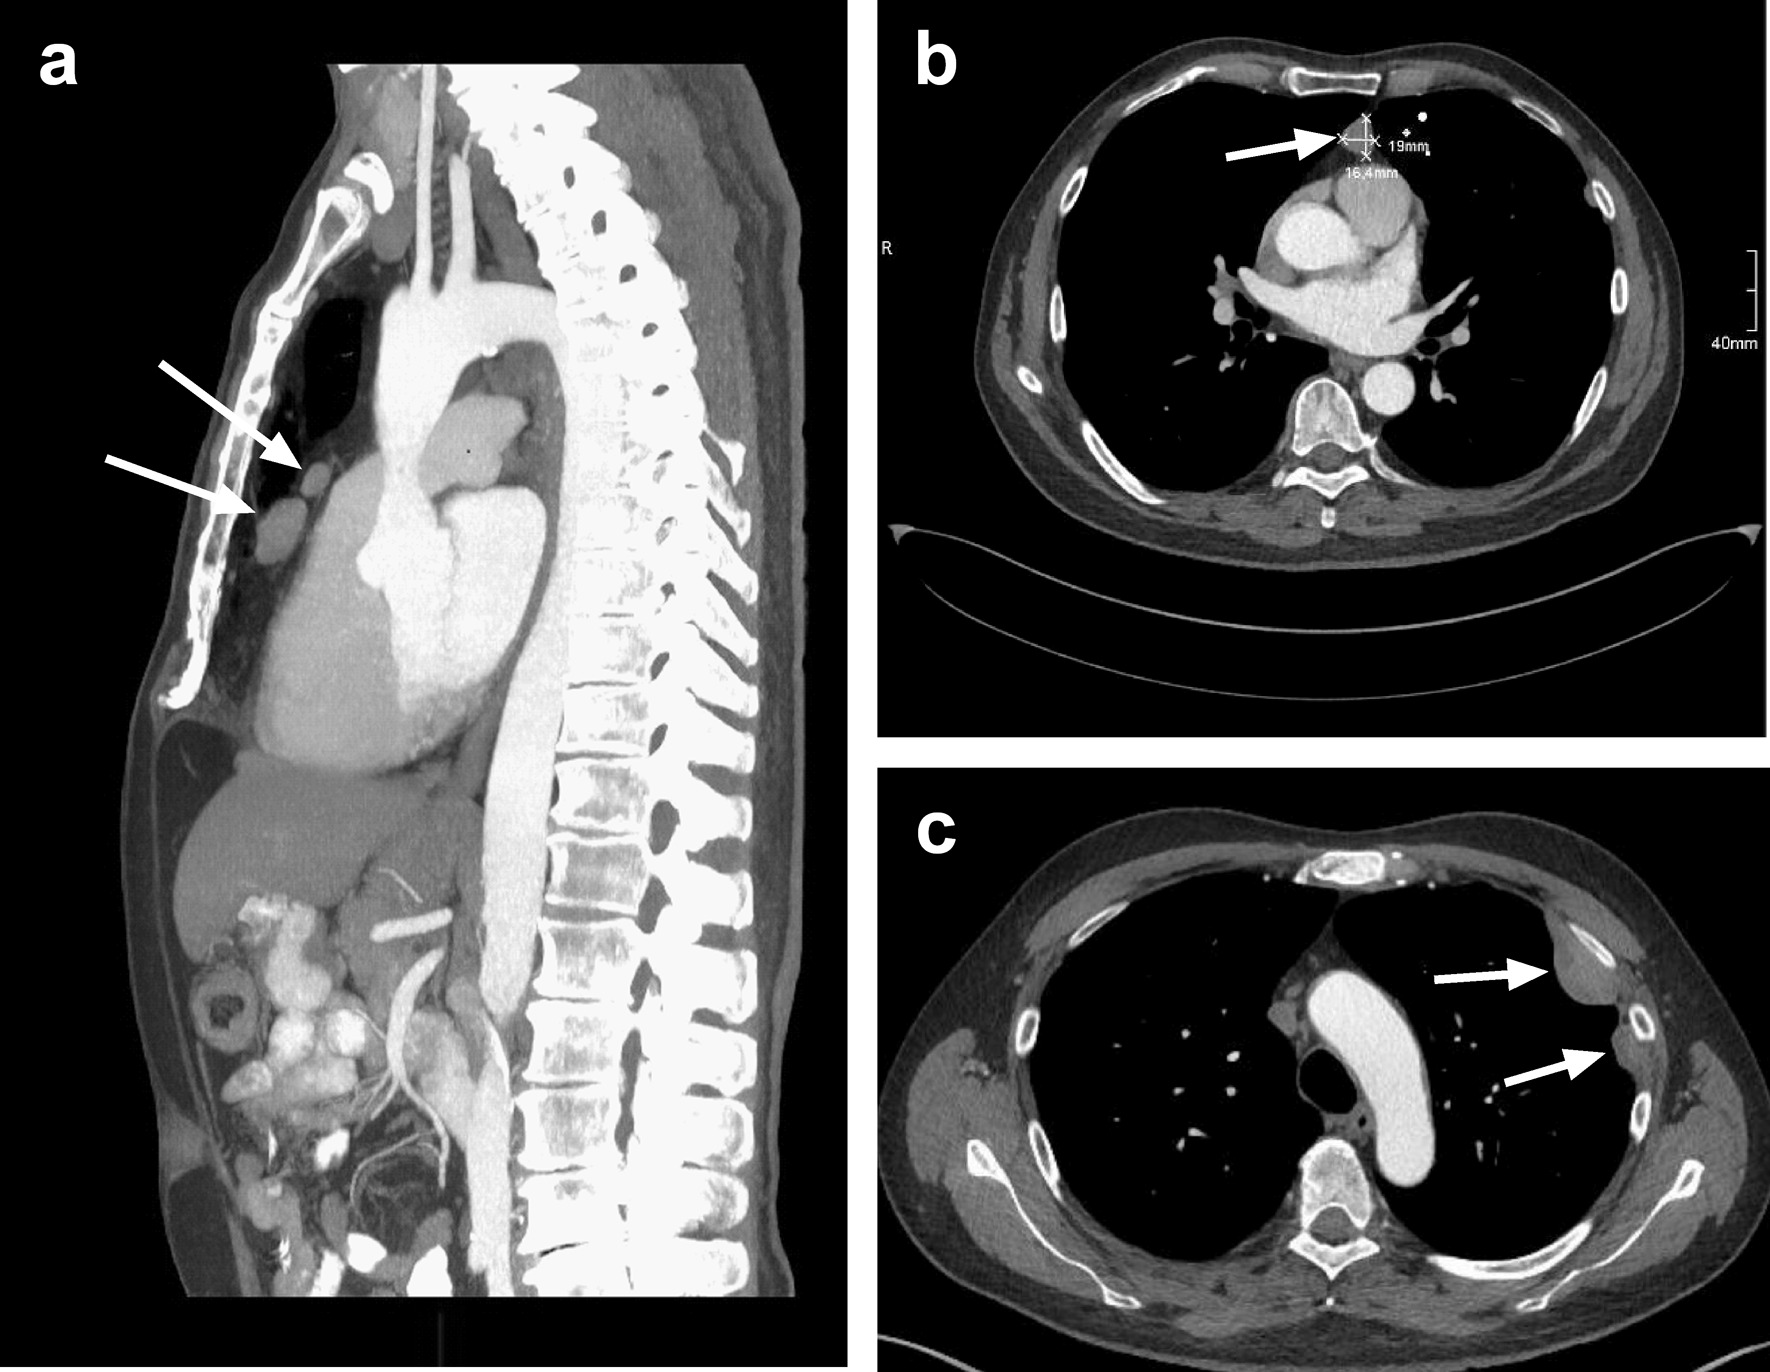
Contrast-enhanced thoracic CT scan (arterial phase). Sagittal (a) and axial planes (b, c) of the thoracic cavity. White arrows indicate masses in the anterior mediastinum (a, b) and at the left costal pleura (c)
